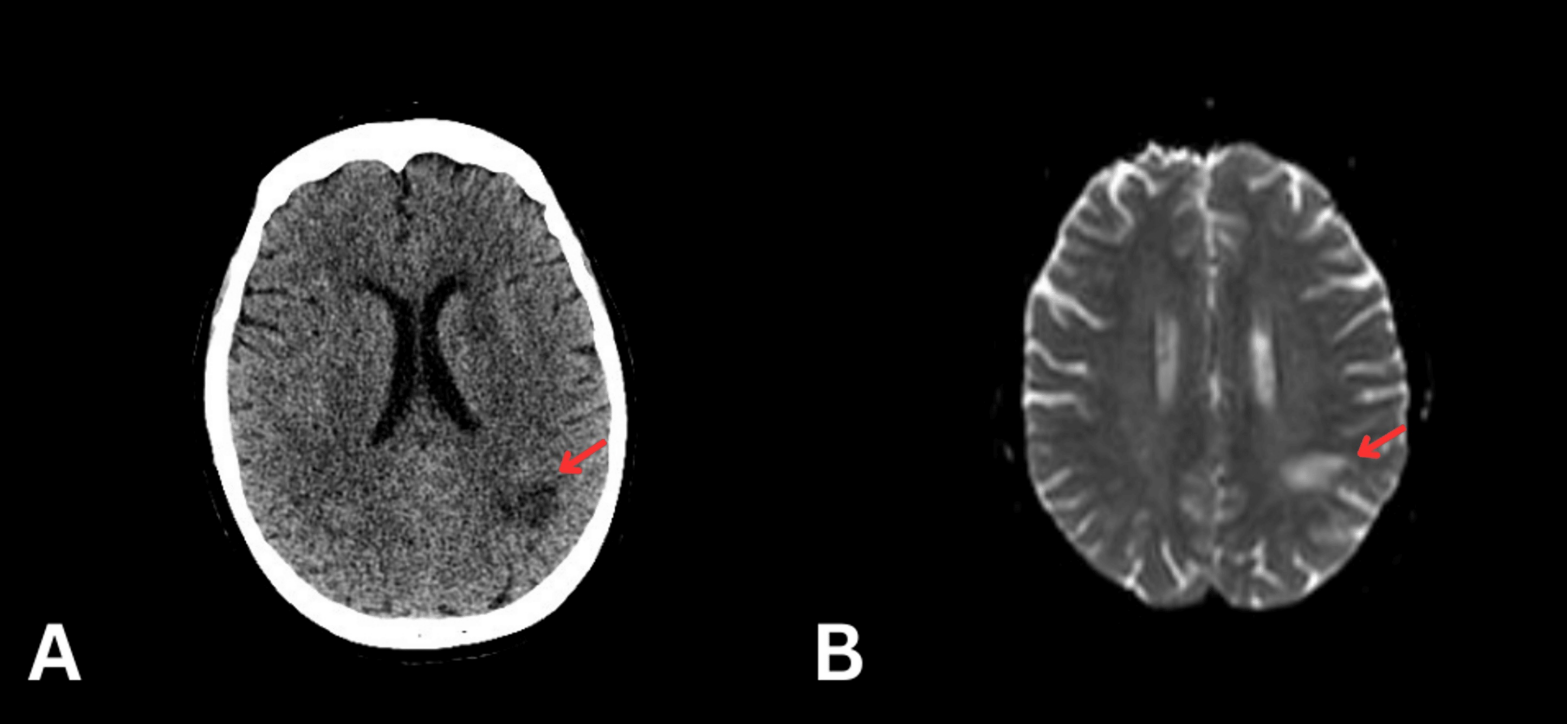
CT head and MRI head. The arrows show the left parietal lobe changes. A. CT head demonstrated left parietal lobe changes, which were considered either demyelination or subacute infarction. B. MRI of the brain confirmed infarction using diffusion-weighted imaging (DWI) and apparent diffusion coefficient (ADC) mapping.
